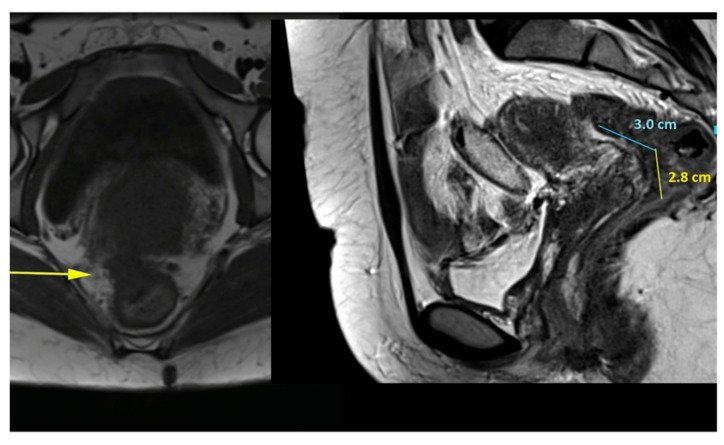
Preoperative MRI image shows deep endometriosis involving the low rectum and vagina.
